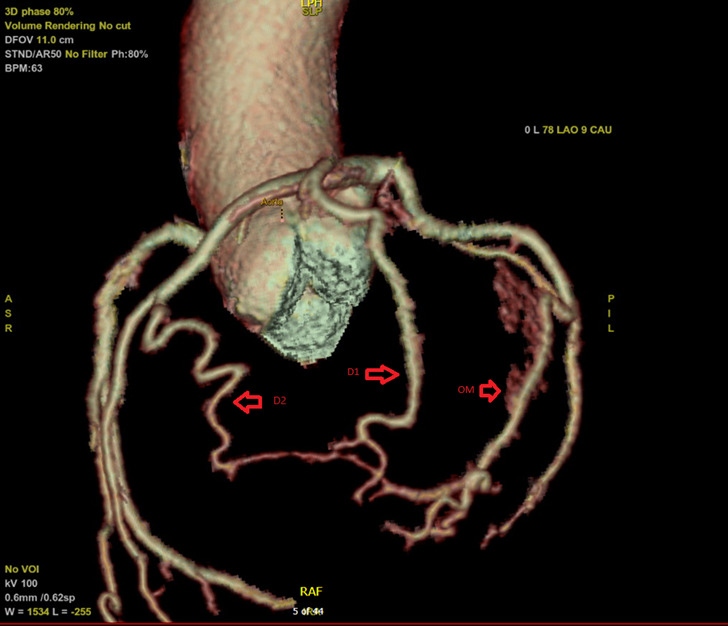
Figure 5. Computed Tomography Angiography showing the connection between the Obtuse Marginal Branch (OM Arrow) of the Left Circumflex Artery with the first Diagonal (D1 Arrow) and second Diagonal branches (D2 Arrow) of the Left Anterior Descending artery.
